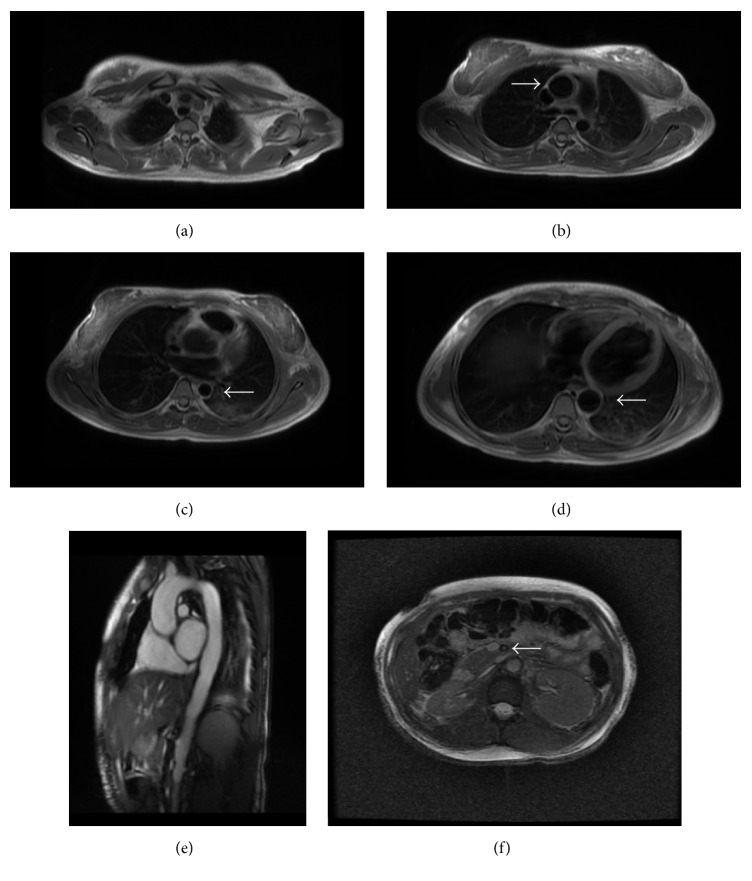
MRI/MRA through the chest, abdomen, and pelvis was obtained. (a) Axial double inversion recovery sequence through the upper chest demonstrates mural thickening of the brachiocephalic, left common carotid, and left subclavian arteries. (b) Axial double inversion recovery sequence demonstrates mural thickening of the ascending aorta which measures 3.4 cm in diameter. (c) Axial double inversion recovery sequence demonstrates a focal narrowing of the descending aorta, measuring 2.2 cm. (d) Inferior to the focal narrowing visualized in (c), the aorta measures 3.2 cm on axial double inversion recovery sequence. (e) Sagittal oblique cine-FIESTA sequence demonstrates the undulating contour of the abdominal aorta. (f) Axial T2 FIESTA fat saturated sequences through the abdomen demonstrate wall thickening and mural narrowing of the superior mesenteric artery. The white arrow represents in (b) ascending thoracic aorta; (c) and (d) descending thoracic aorta; (f) SMA.
